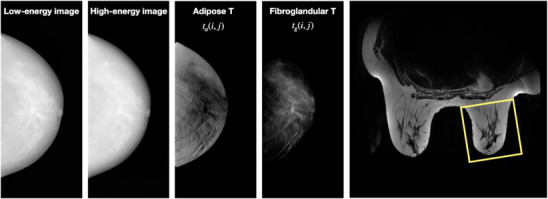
From left to right: LE and HE “for processing” mammograms, adipose tissue map, fibroglandular tissue map, MRI slice with a ROI delineating the analyzed breast (yellow rectangle). The measured VBD was 24.6% in mammograms and 29.0% in MRI. Hyper‐intense regions inside the MRI volumes of interest were considered adipose tissue.
